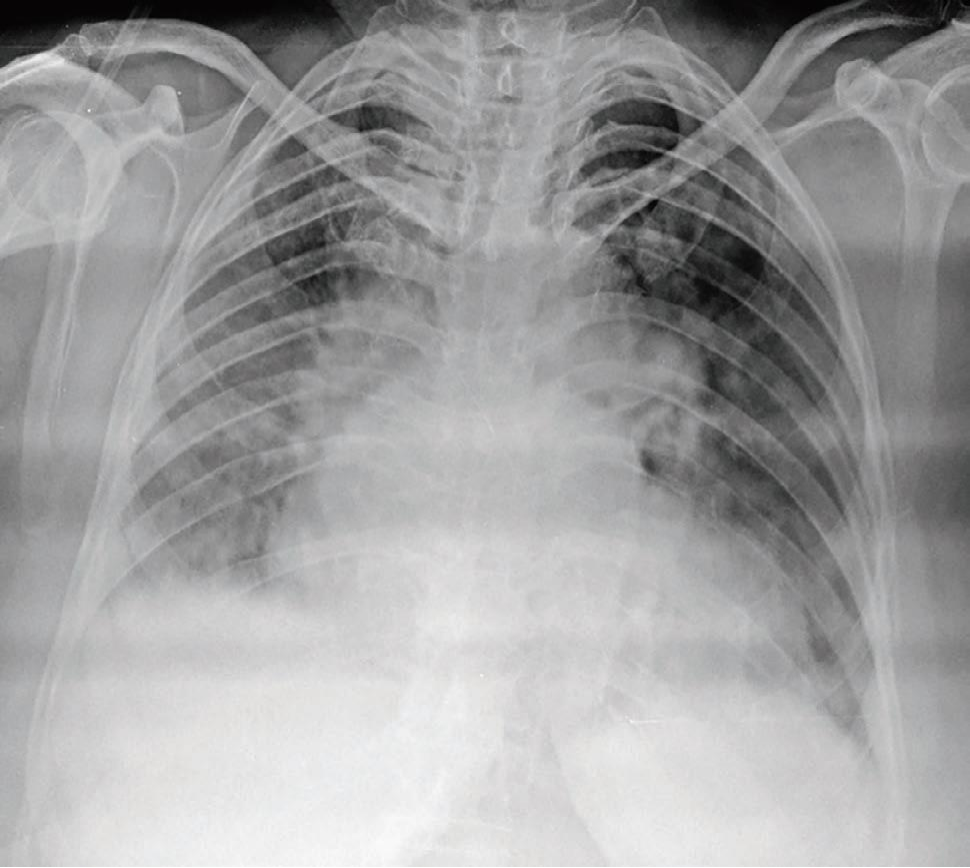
Chest radiograph (AP view) shows cardiomegaly, prominent hilar shadows, and diffuse haziness in lung fields. No significant loss of lung volume/mediastinal shift seen.
